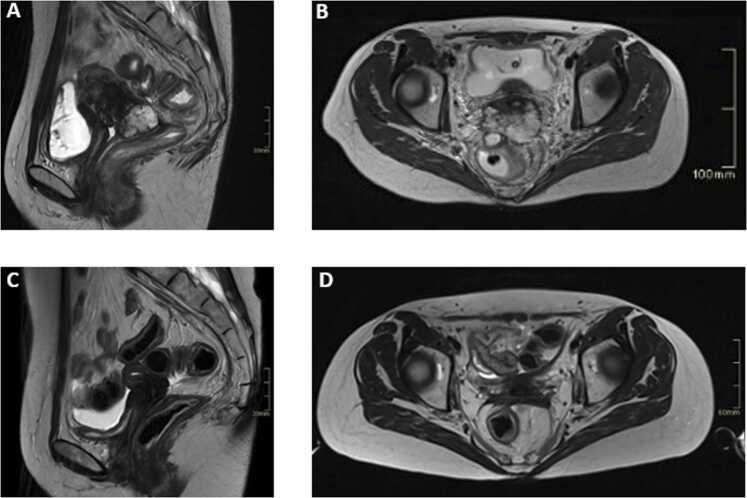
Magnetic resonance imaging (MRI) of the pelvic lesion before and after carbon ion radiation therapy (CIRT) boost. (A) and (B) Pretreatment MRI in the sagittal (A) and axial (B) planes shows a pathological mass centered at the level of the uterine cervix, infiltrating the posterior vaginal fornix and the posterior wall of the upper third of the vagina. The lesion demonstrates multilobulated margins with nodular extensions, including an anterior nodule invading 15 mm into the right parametrium. Posteriorly, the mass infiltrates the anterior perirectal fat tissue with a distance of 2 mm from the rectum. An exception is noted for a pseudo-nodular extension that comes into close contact with the anterior wall of the proximal rectum, with no clearly discernible intervening fat plane. (C) and (D) Post-treatment MRI in the sagittal (C) and axial (D) planes obtained 6 months after the CIRT boost demonstrates a complete response.
